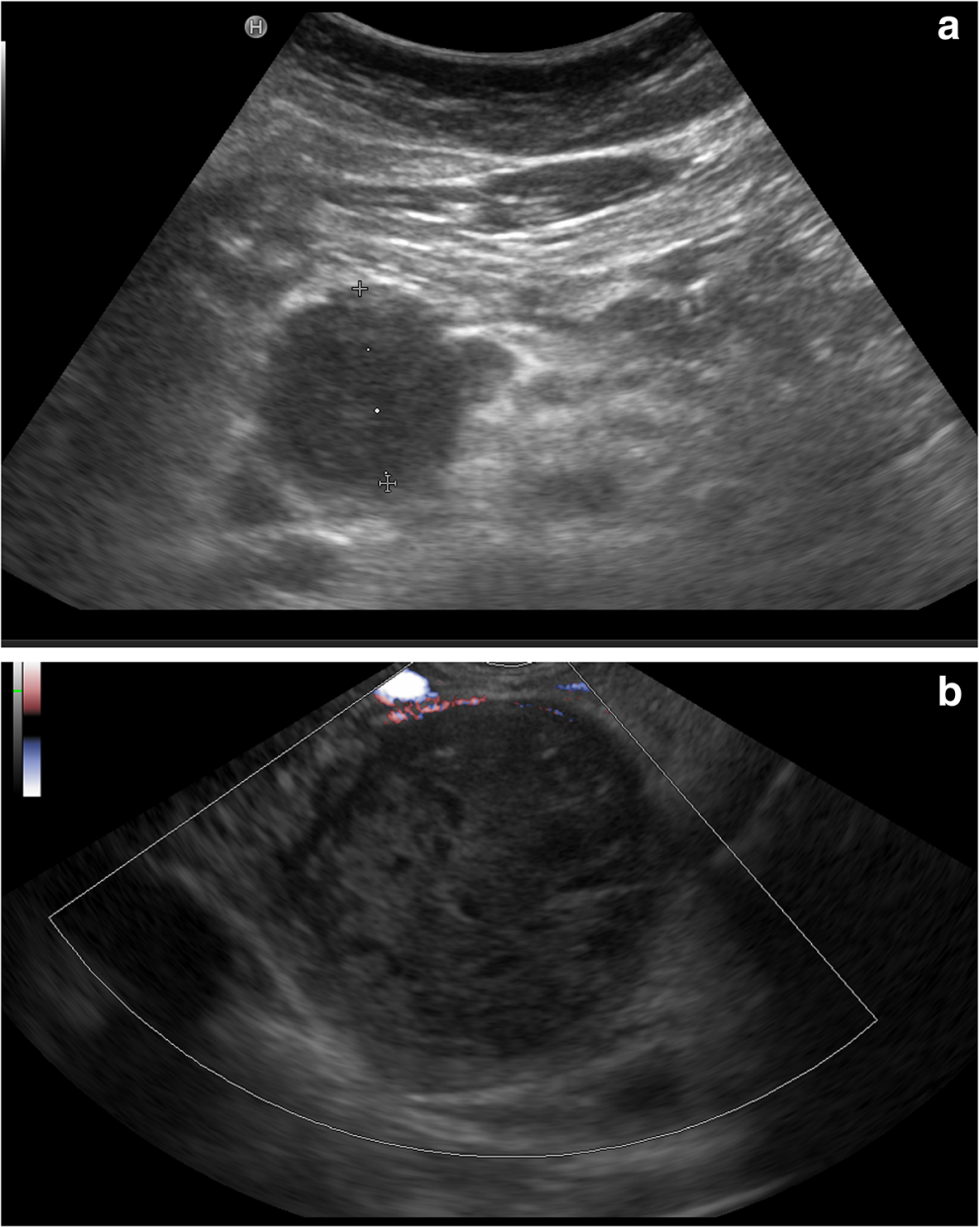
Findings from ultrasound sonography. a trans abdominal ultrasound sonography showing a mass in the pancreatic caput. b endoscopic sonography of the pancreas confirmed a large mass in the head of the pancreas
